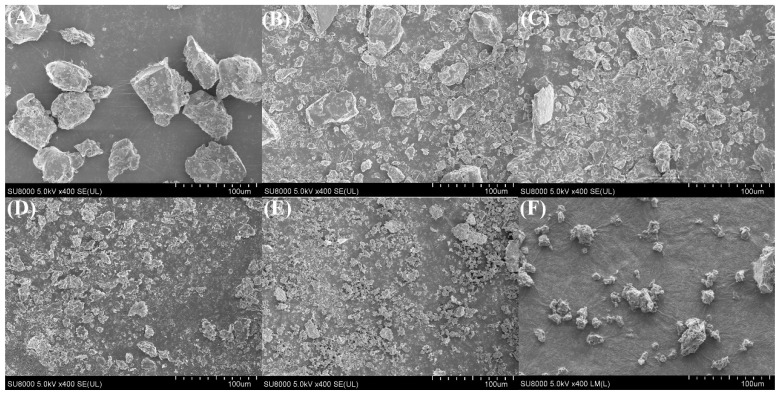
Effect of ultrasonic intensity on the change in SEM apparent morphology of CPP protein during ultrasound-assisted acidic extraction, where A–E represent samples with ultrasonic intensities: (A) 0 W/cm^2^, (B) 3.03 W/cm^2^, (C) 6.07 W/cm^2^, (D) 9.10 W/cm^2^, and (E) 12.13 W/cm^2^; (F) 15.16 W/cm^2^.
